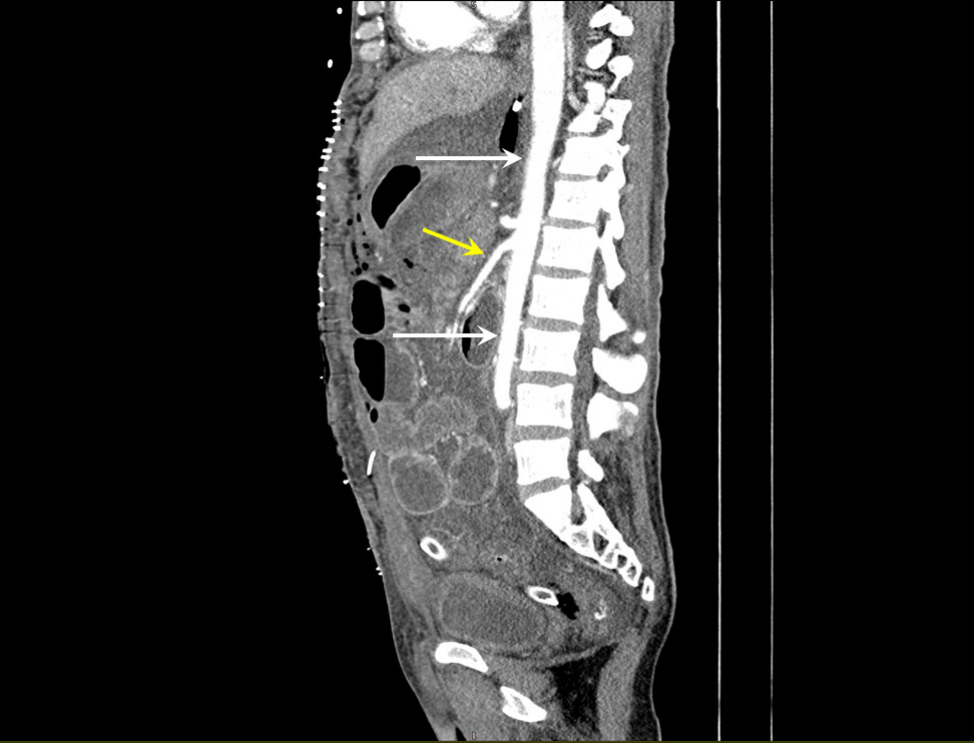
Sagittal slice of an arterial phase CT scan, demonstrating return to normal opacification of the abdominal aorta (white arrows) and the superior mesenteric artery (yellow arrow).
